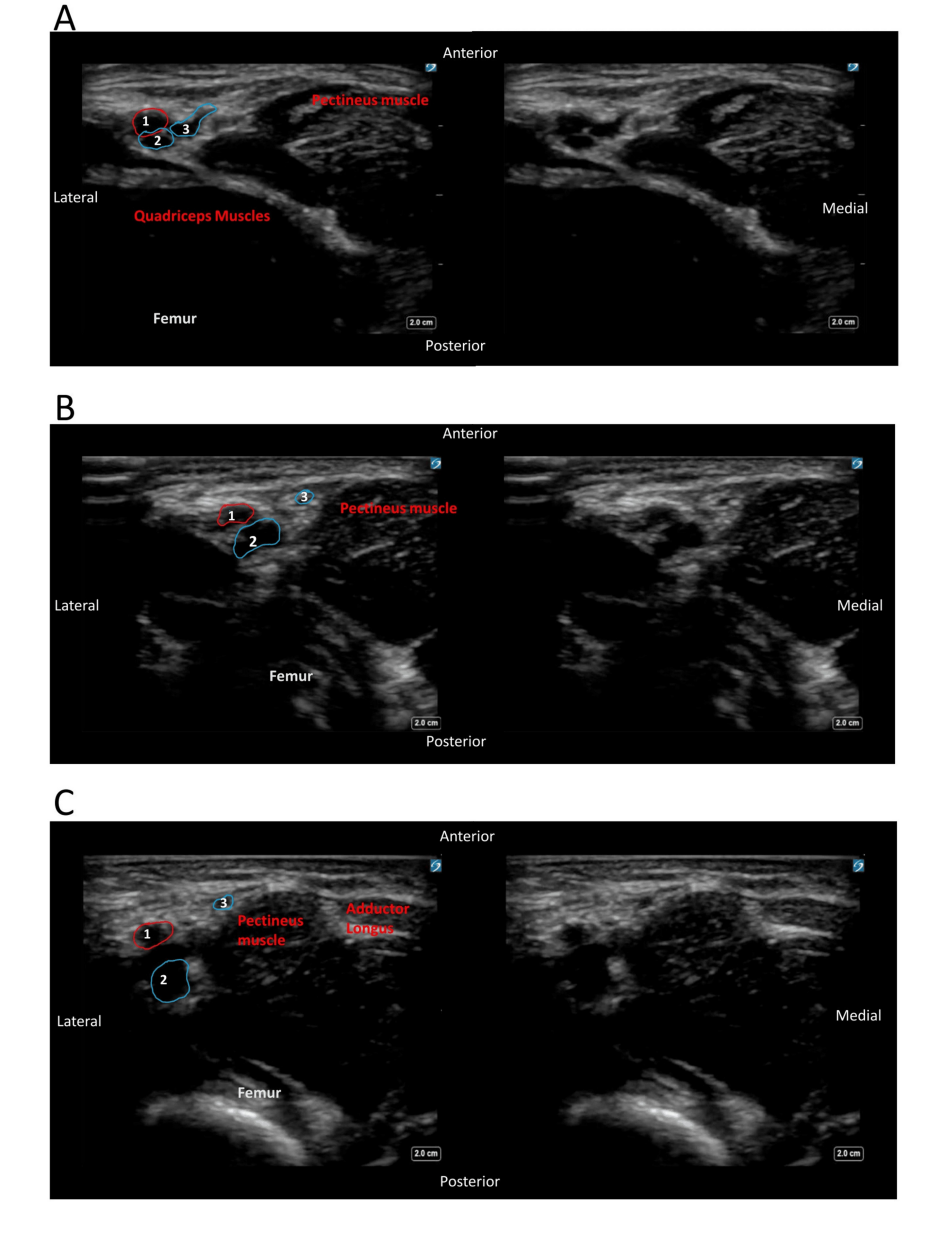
Ultrasound images of the vessels in the right femoral triangle in a proximal-to-distal sweep in the axial plane, with an annotated image on the left and original image on the right (A) Most proximal view demonstrating branching of the GS vein from the common femoral. (B) Middle view of the sweep with the GS separating from the femoral vein superficial to the pectineus muscle. (C) Distal view of the GS separation from the femoral vein, traversing superficial to the pectineus muscle with the adductor longus — medial border of the femoral triangle — coming into view. 1: femoral artery; 2: femoral vein; 3: GS vein GS: greater saphenous
